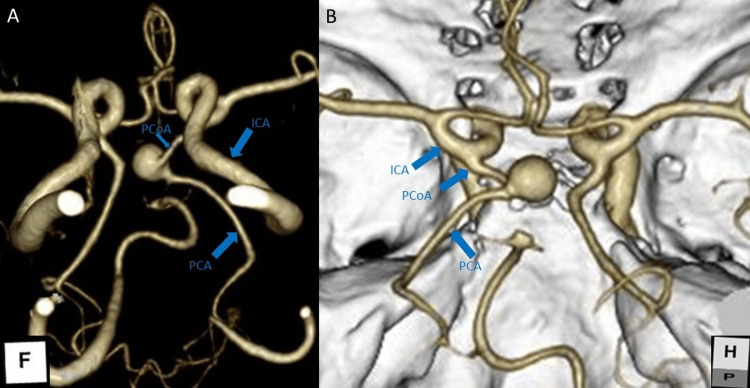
Computed tomography angiography (CTA) of the brain. (A) CTA image showing a saccular aneurysm arising medially from the left PCoA. (B) Fetal-type configuration of the left PCoA is observed, with an absent P1 segment of the PCA. ICA, internal carotid artery; PCoA, posterior communicating artery; PCA, posterior cerebral artery
